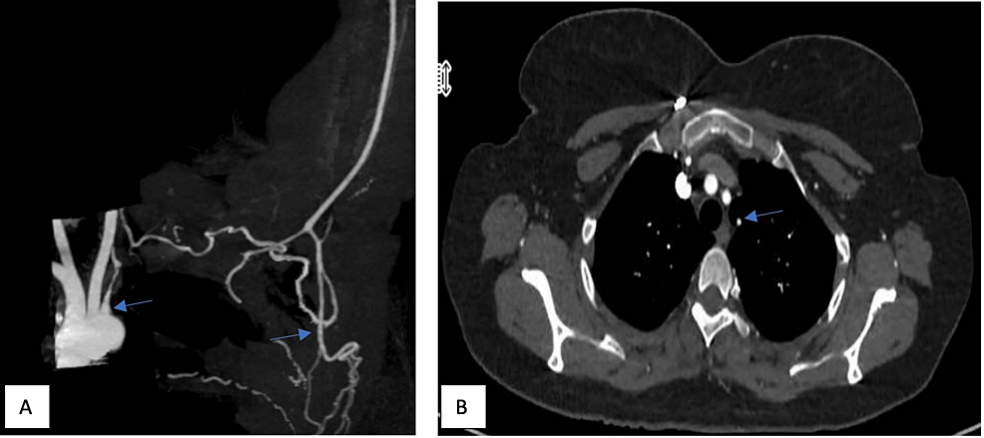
Maximum intensity projection (MIP) coronal image (A) and axial thin cuts (B) of CT angiography in Case 13 showing complete obliteration of the left subclavian artery after the origin of the left vertebral artery (arrow) with severe attenuation of the proximal left subclavian artery and vertebral artery; (A) Reconstitution of the left upper limb arterial supply from the cervicothoracic collaterals from the axillary artery onwards
